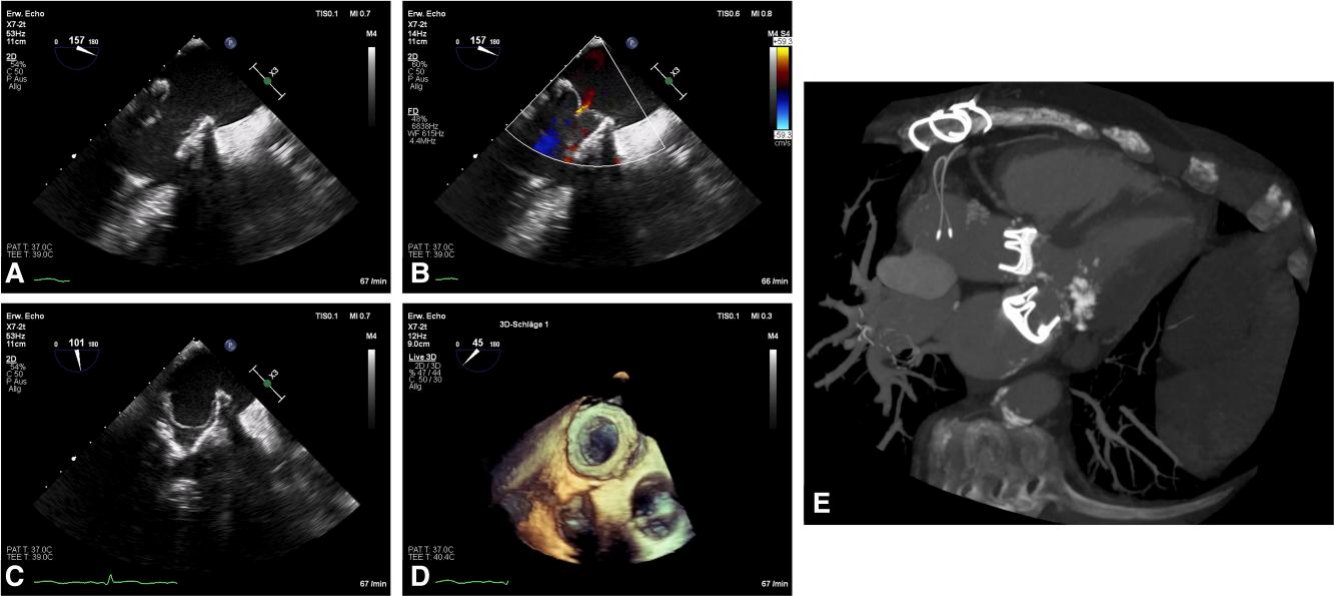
Echocardiographic findings depicting the intra-atrial implantation of a Dacron collar carrying a mitral bioprosthetic valve (*A–D*). Computed tomography scan showing the prosthetic aortic valve in its usual position, and the intra-atrially placed MPV above a severely calcified mitral annulus (*E*). MPV, mitral bioprosthetic valve; MAC, mitral annulus calcification; APV, prosthetic aortic valve.
